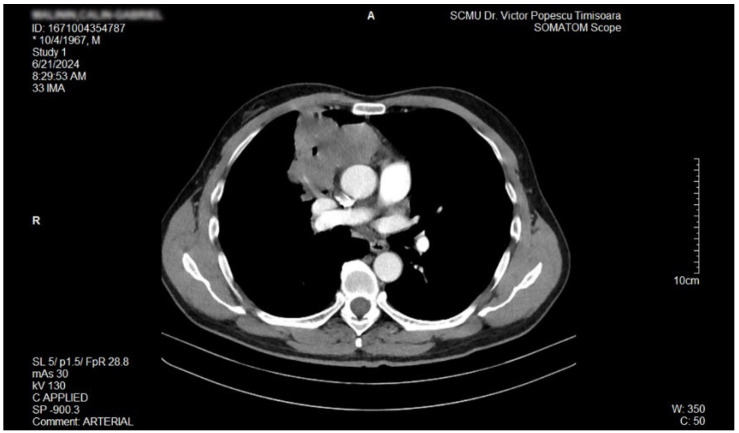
Contrast thorax CT: large right midthoracic tumor with mediastinal extension.(axial view.
